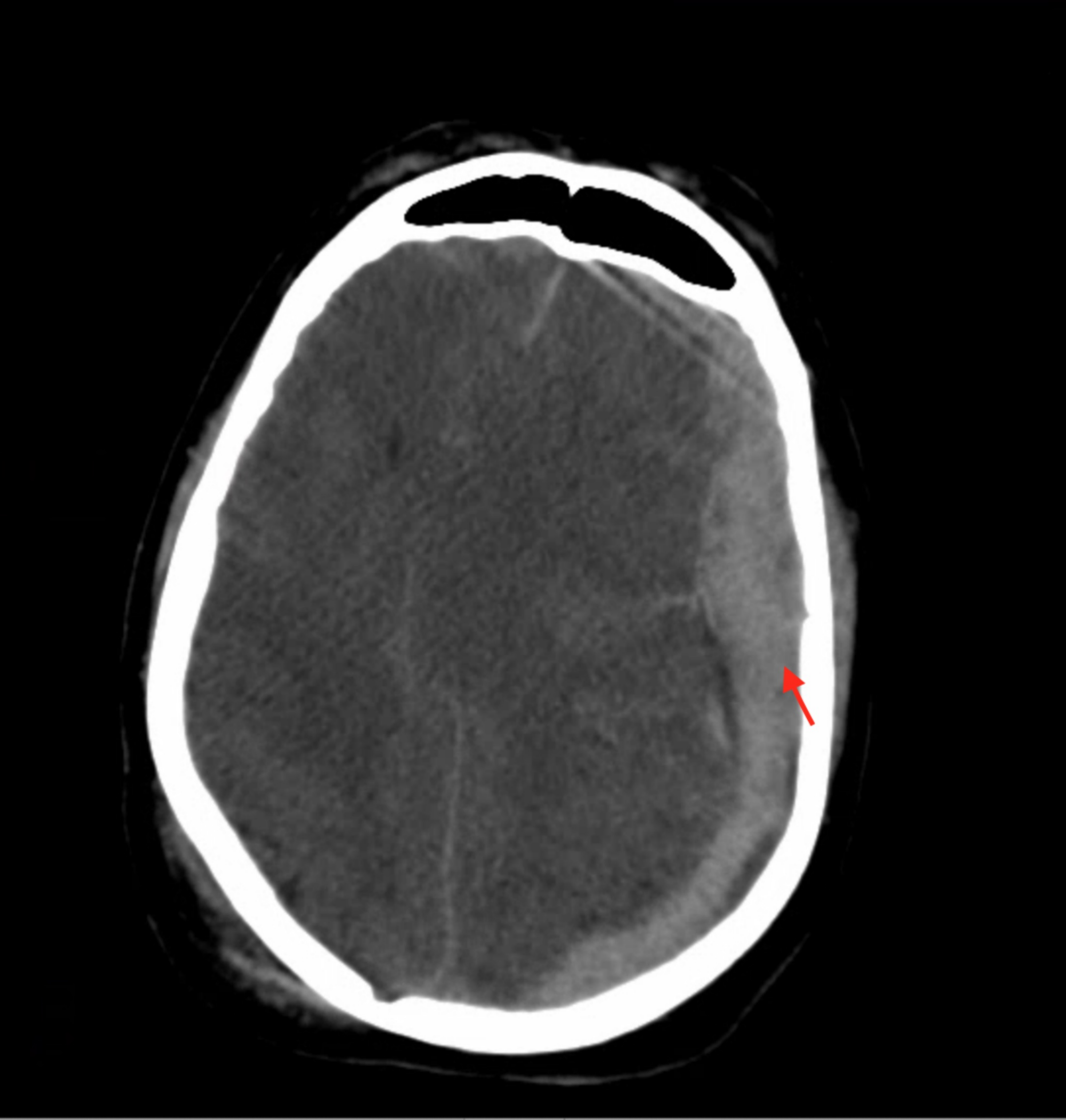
Computed tomography of the head demonstrating initial 22 mm traumatic subarachnoid hemorrhage with a 21 mm of midline shift
